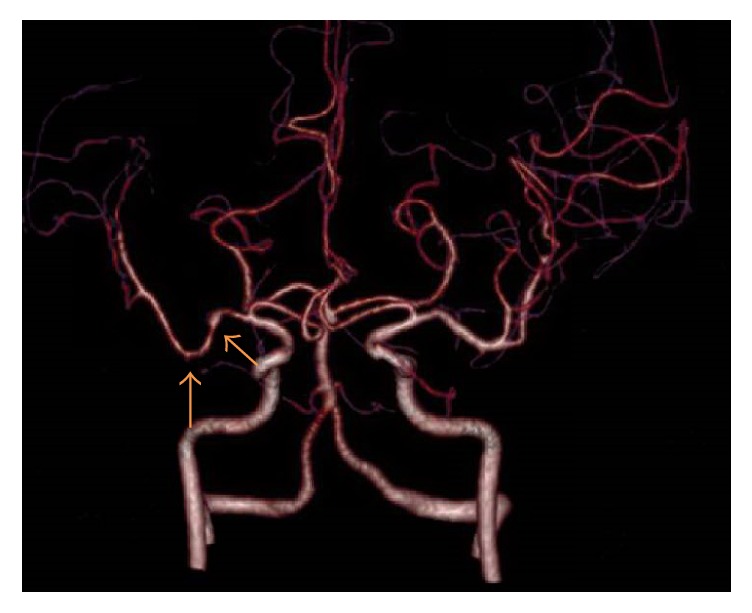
CT angiogram of the circle of Willis showing multiple areas of luminal irregularity.
